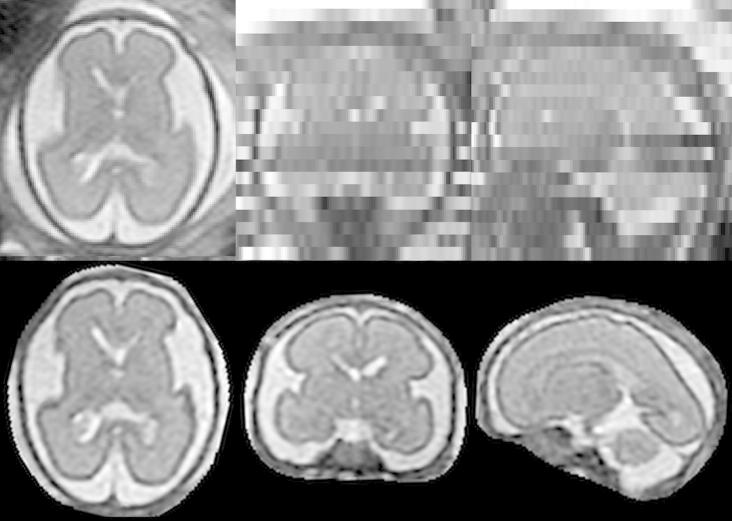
Reconstructed fetal brain MRI of a subject with GA 26 weeks using only five stacks of slices. First row: One of the acquired stacks. Second row: Reconstructed volume.
